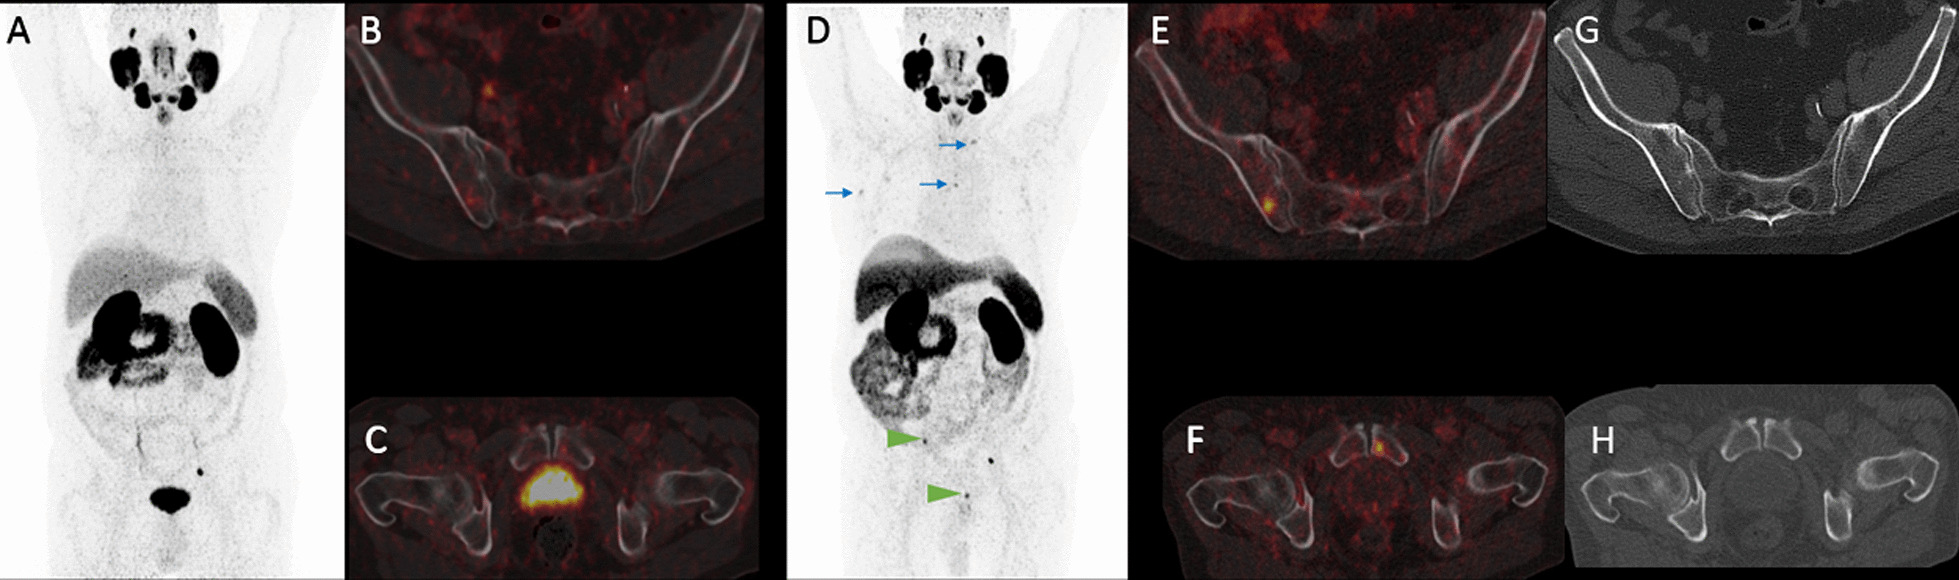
MIP of [^68^Ga]-Ga-PSMA-11 PET (A) with obvious focal uptake only paraureteral left. In axial fused PET/CT of the iliosacral region (B) and the symphysis (C), no focal uptake suggestive for malignancy is present. The MIP of [^18^F]-F-PSMA-1007 (D) shows beside the concordant paraureteral uptake on left side intensive focal uptake in the right iliac bone (SUV_max_: 16.5) and the left pubic bone (SUV_max_: 14.8 arrow heads). Axial fused PET/CT confirms the intraosseous location of both the iliac (E) and the pubic metastasis (F). While the right iliac lesion has a slight hypersclerotic correlate in CT-imaging (G), there is no morphologic correlate in the left pubic bone detectable in low-dose CT (H). Auxiliary findings in [^18^F]-F-PSMA-1007 (D): presumable unspecific uptake in cervical ganglia, mediastinal and axillary lymph nodes (blue arrows) as well as multifocally in the rib thorax
